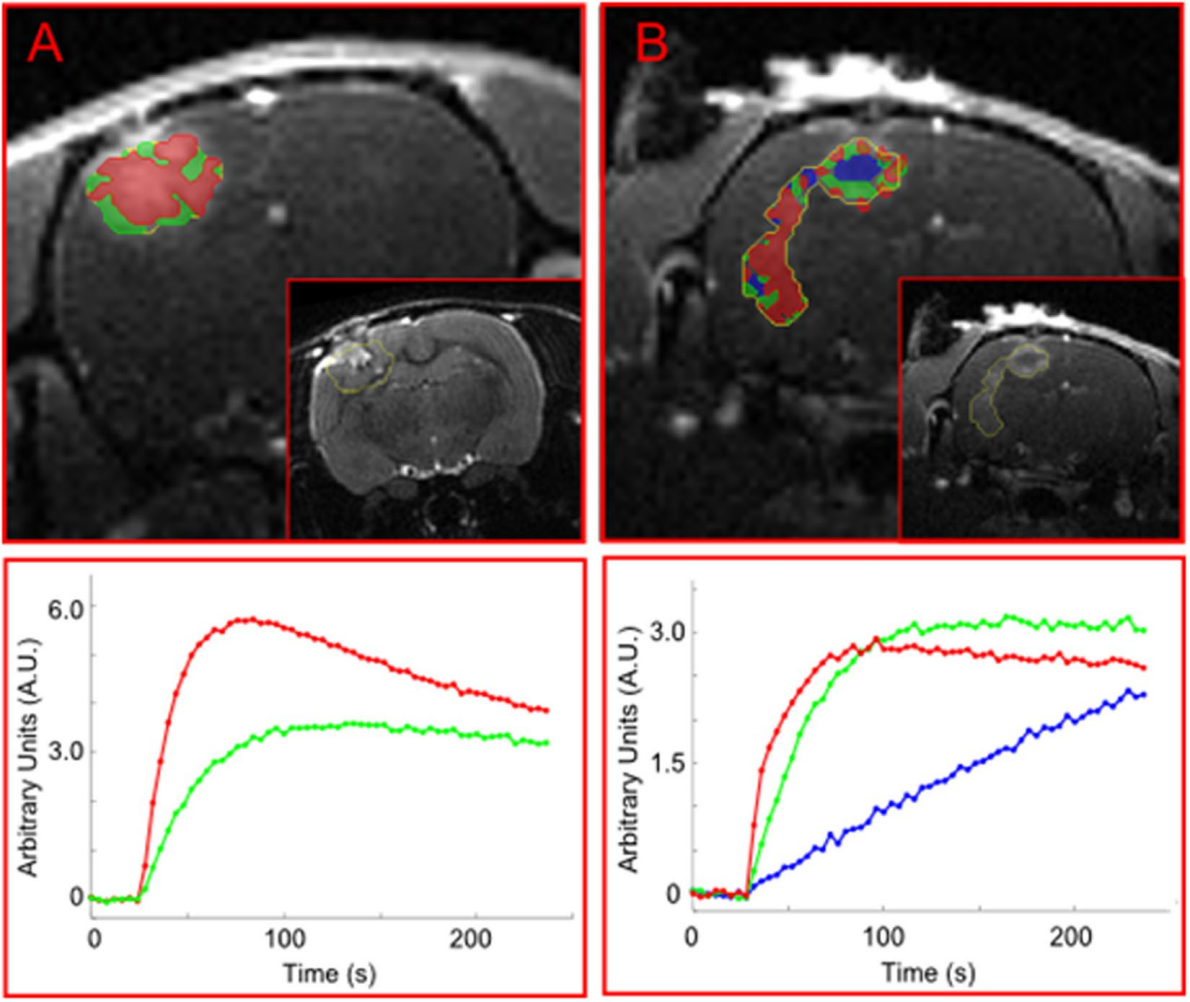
Mapping of tumor habitats in a pre-clinical brain tumor model. (A,B) Representative coronal slices with mapped tumor habitats in DCE-MRI data from pre-clinical models of brain tumors (left (A): U87, right (B): RG2). (Insets) Corresponding anatomical images with outlined tumor volume. Automatic determination of the number of signal-related PCs revealed correspondingly two and three signal-related PCs; (Bottom) Corresponding characteristic average signal-*versus*-time curves from each habitat. Red, green, and blue well-perfused, hypoxic, and necrotic habitats respectively.
